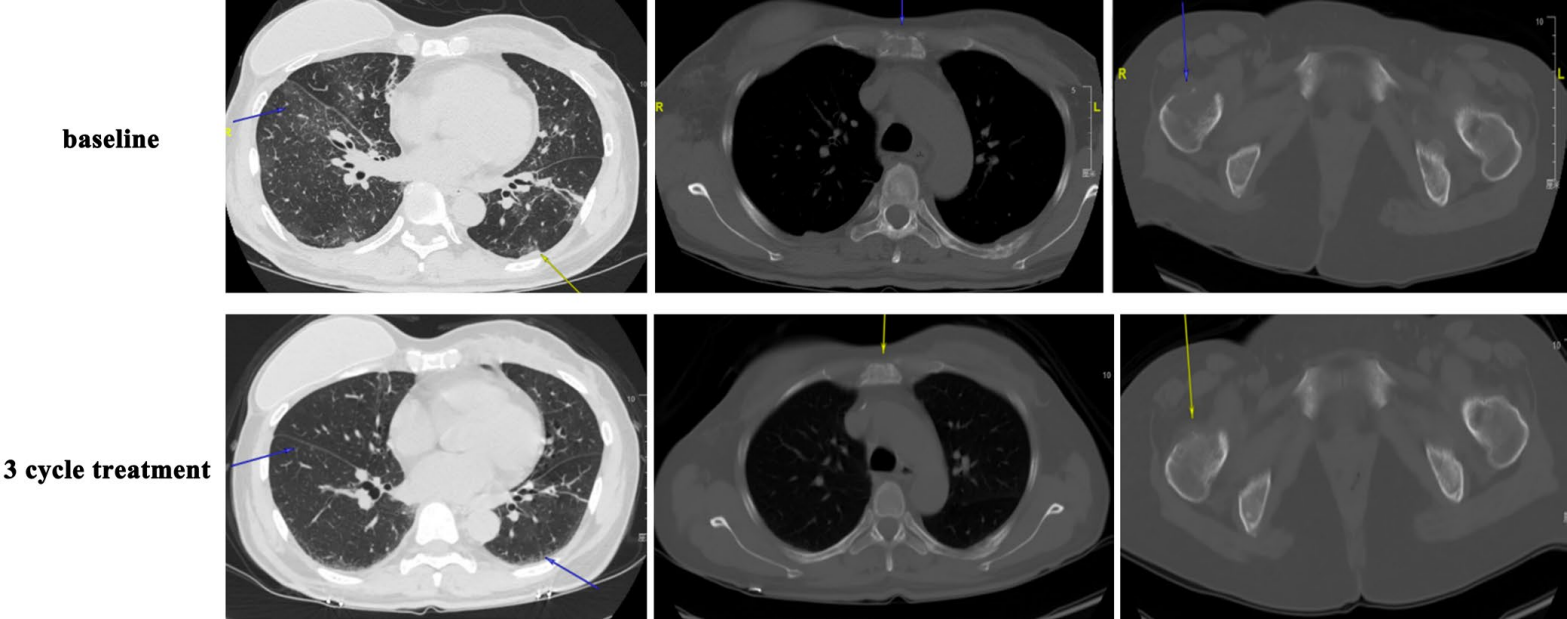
CT evaluation after first‐line THP treatment.
